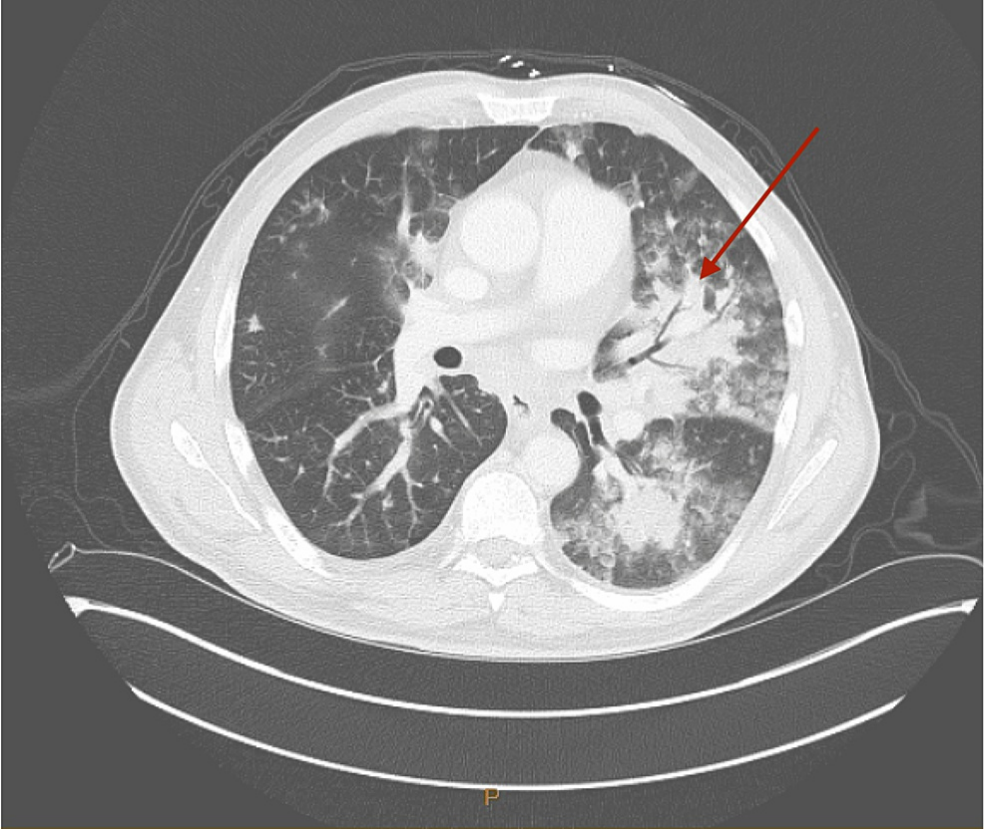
CT chest with contrast showing extensive left greater than right nodular airspace opacities and air bronchogram (arrow) consistent with multifocal pneumonia
